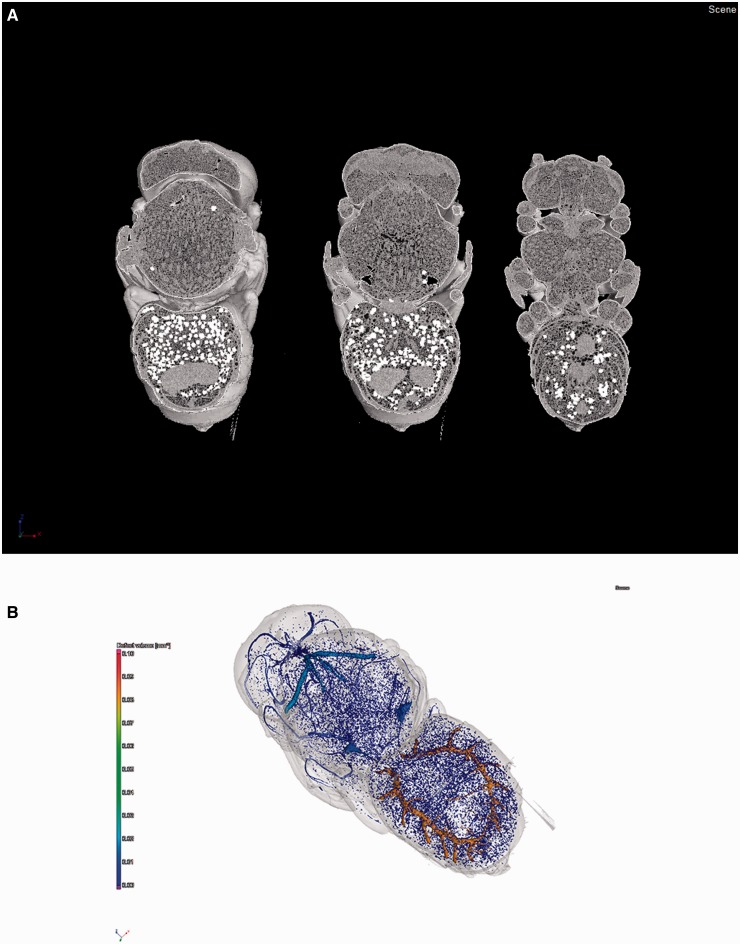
Micro-computed tomography images of a pupal alfalfa leafcutting bee, *Megachile rotundata*, 14 days after metamorphosis was initiated. (A) Digital cross-sections of the bee after imaging and reconstruction. (B) Artificially colored image showing air-filled tracheae that were identified after reconstruction. Color scale indicates volume of identified tubes.
